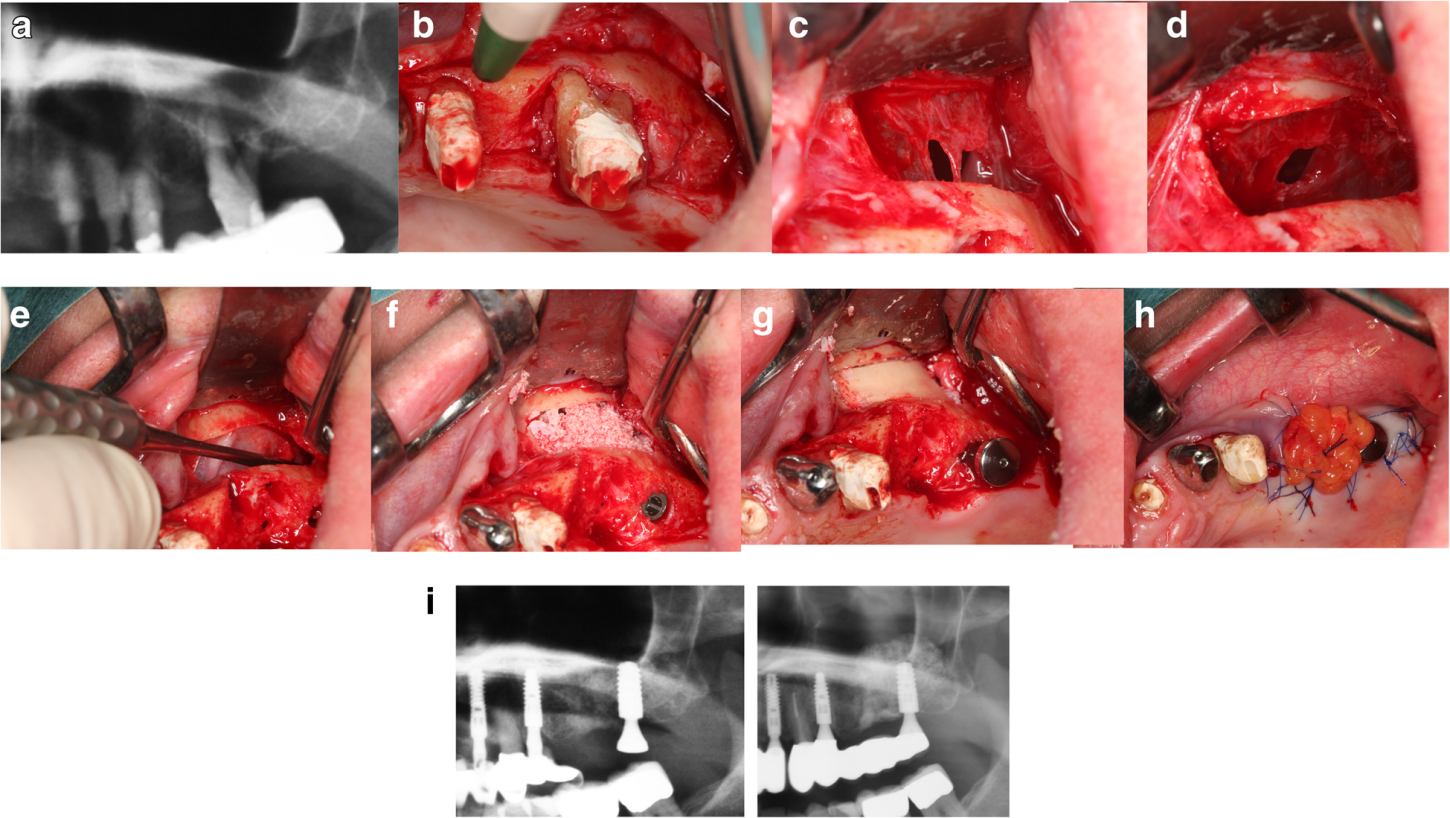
a Pre-operative X-ray. b Tooth 26. c Perforation of the Schneiderian membrane. d Perforation after elevating the Schneiderian membrane. e Covering the perforation with a collagen membrane and fibrin glue. f Augmentation and implant inserted. g Repositioning of the buccal bone. h Covering of the OAC with the BFP. i Post-operative X-ray vs 3 years post
